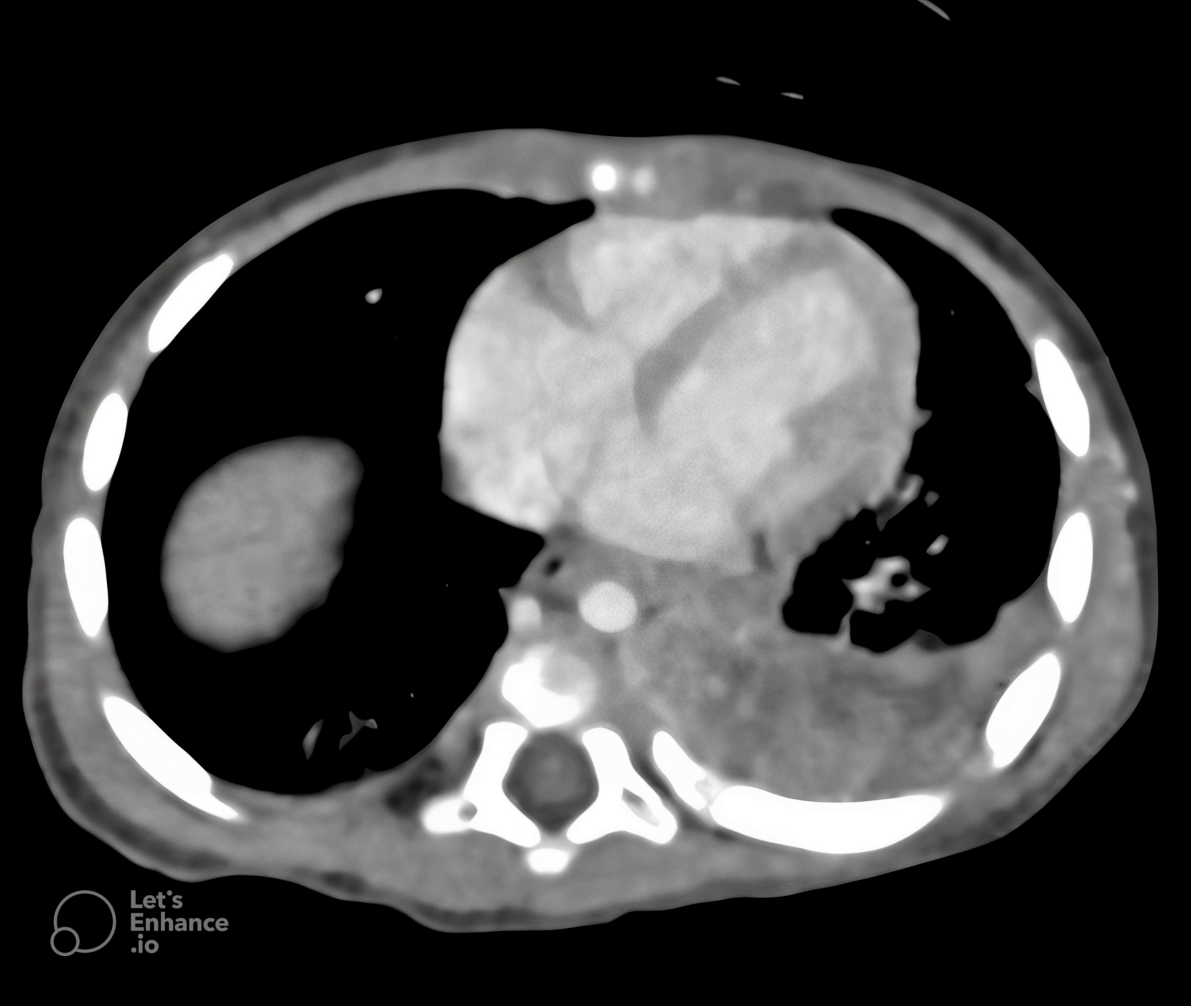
A follow-up chest CT, performed 14 days after initiating VAC chemotherapy, demonstrates a remarkable reduction in tumor size. VAC: Vincristine, Actinomycin D and Cyclophosphamide.
